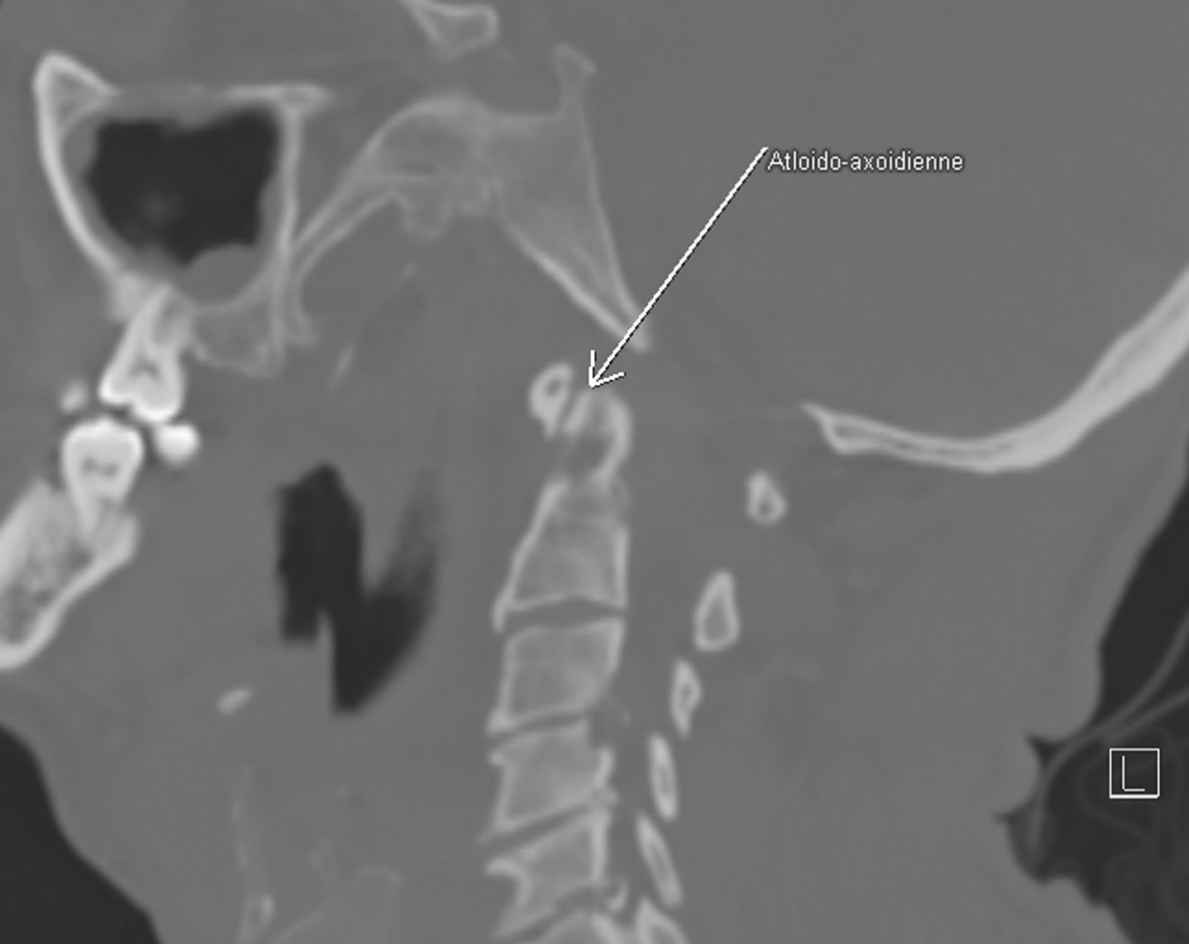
Osteolysis in synovial fold zone of the atlantoaxial articulation in CT scan
